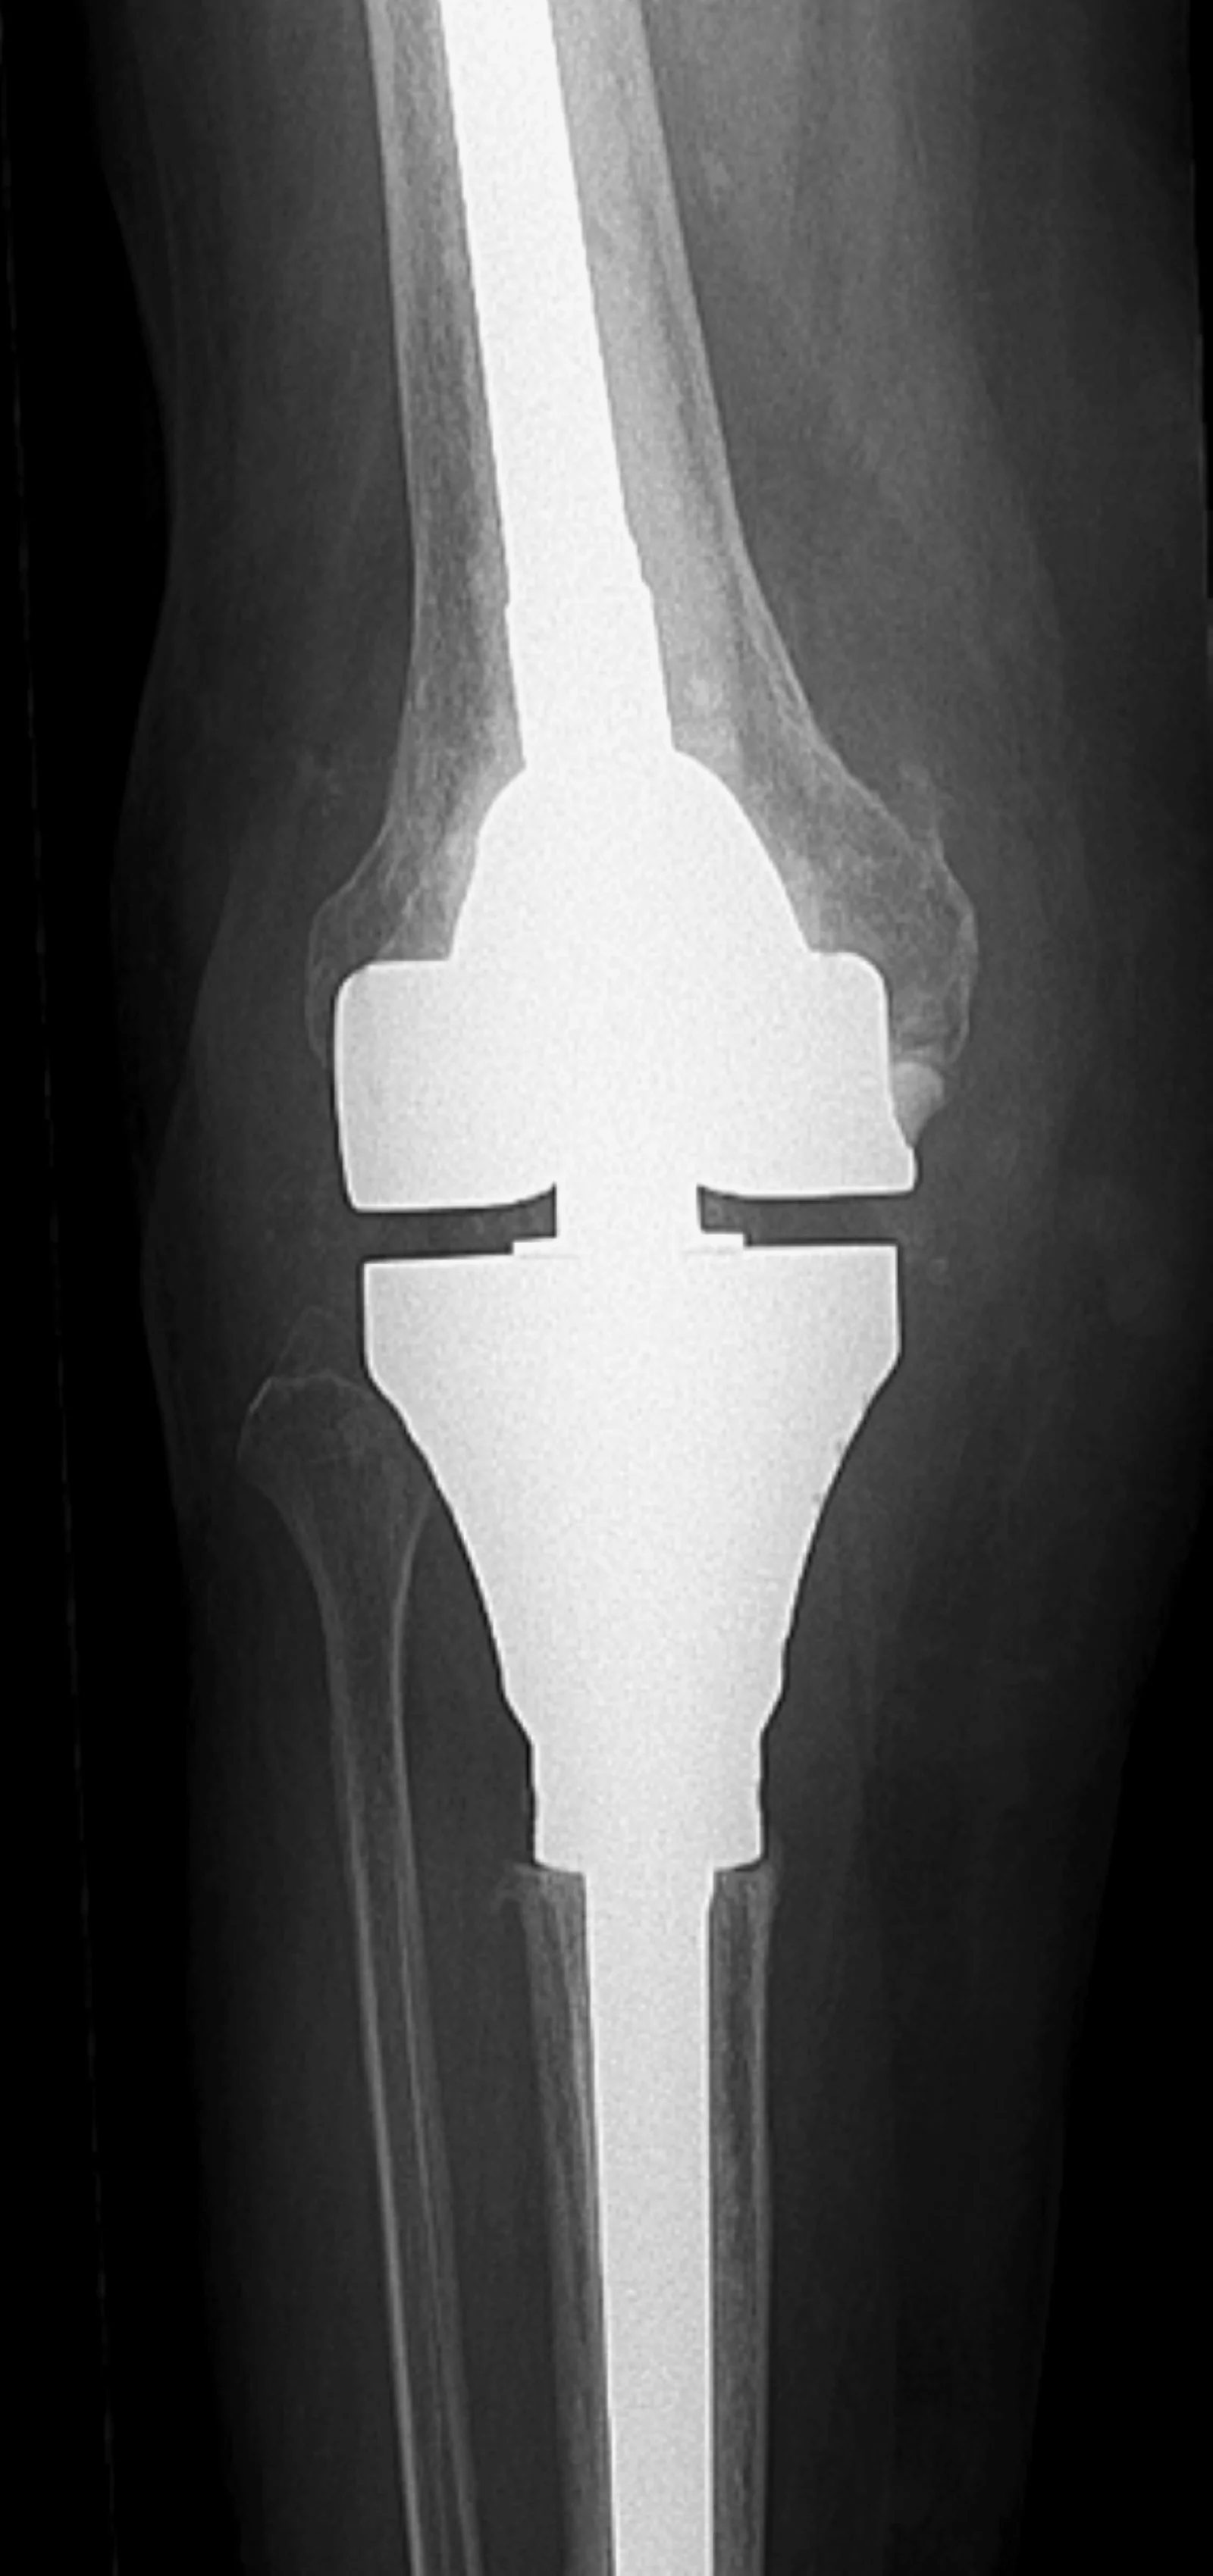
Anteroposterior radiograph of the right knee one year postoperatively showing appropriate implant positioning and no complications after revision arthroplasty.
